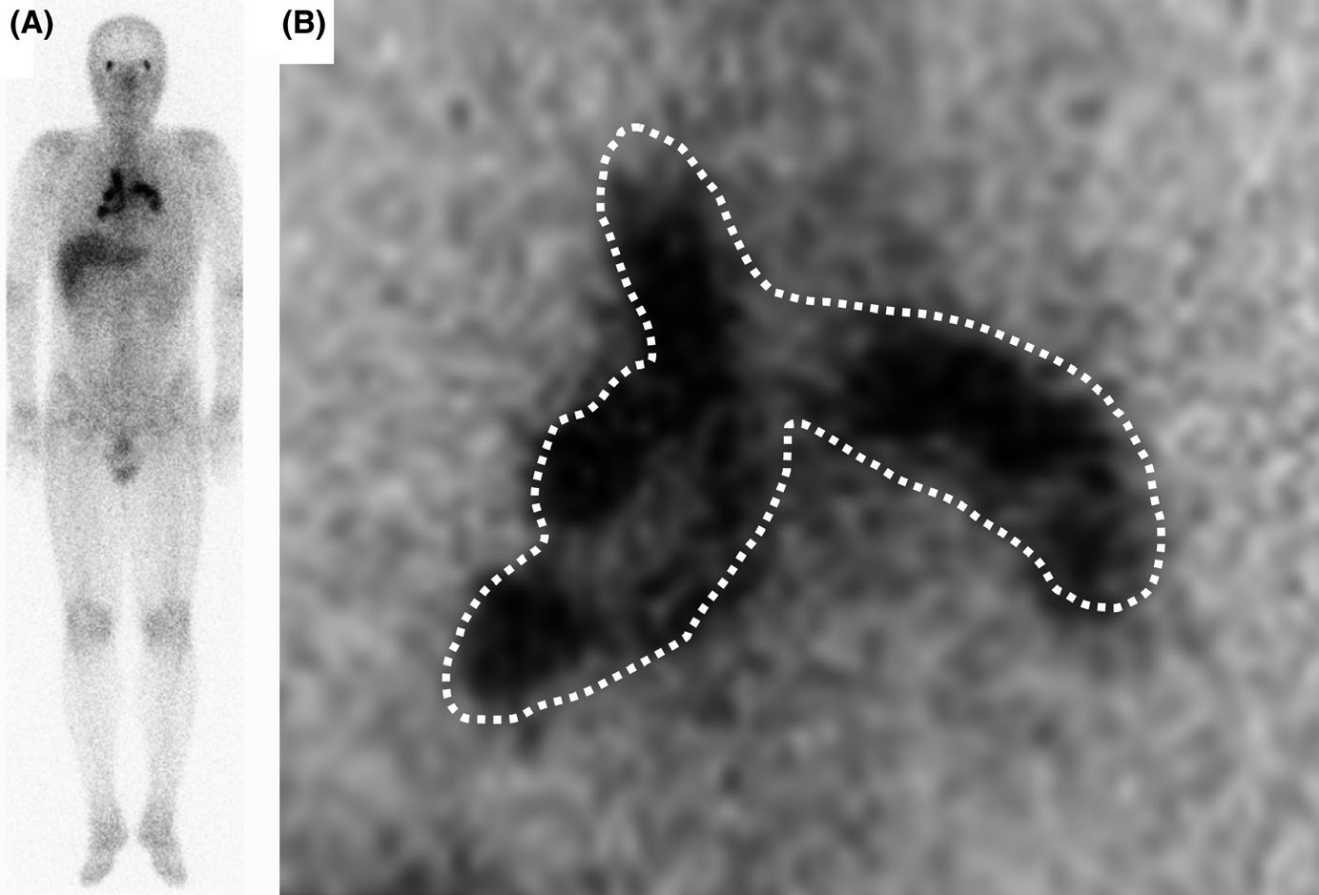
Gallium‐67 scintigraphy. (A) Gallium‐67 scintigraphy shows abnormally high uptake of gallium‐67 in lymph nodes at bilateral hila and mediastinum, and along bronchovascular bundles, which is typical lambda sign, in addition to abnormal uptake in both lachrymal glands. (B)Abnormally high uptake looking like Greek alphabet “λ,” is indicated with dotted line
